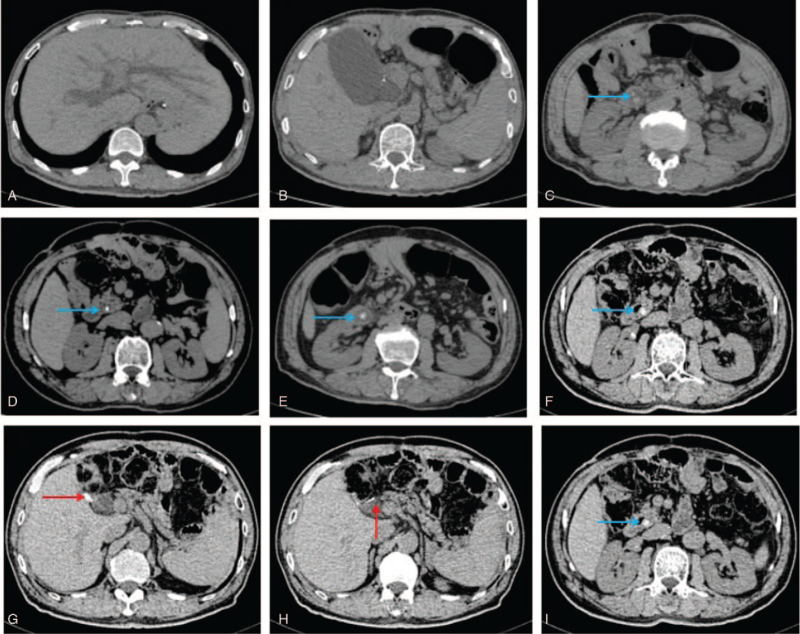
Computed tomography findings. (A) Computed tomography before the first laparoscopic common bile duct exploration (LCBDE) showing a dilated intrahepatic bile duct. (B) Enlarged gallbladder with a thickened wall. (C) Dilated common bile duct (1.5 cm in width) with stones in the distal aspect (blue arrow). Extensive adhesions were present under the incision. (D) A calcified shadow (1.0 cm in length) was present 6 months after LCBDE without symptoms. (E) A circular low-density shadow around the calcified shadow 9 months after the previous operation. (F) The calcified shadow enlarged to a diameter of 1.0 cm 12 months after the LCBDE. (G, H) Three clips placed (red arrow): 2 clips were placed on the cystic duct and 1 clip was placed on the cystic artery; dilation of the common bile duct is noted (1.5 cm in width). (I) A calcified shadow (1.0 cm in length) in the distal common bile duct (blue arrow).
